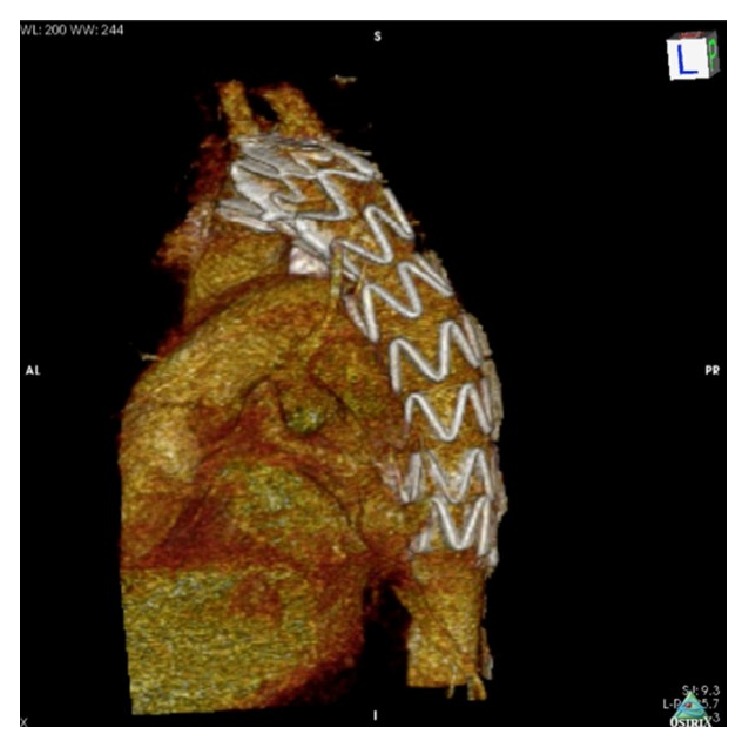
Thorax CT angiography showed good apposition of thoracic stent graft with complete exclusion of PDA.
